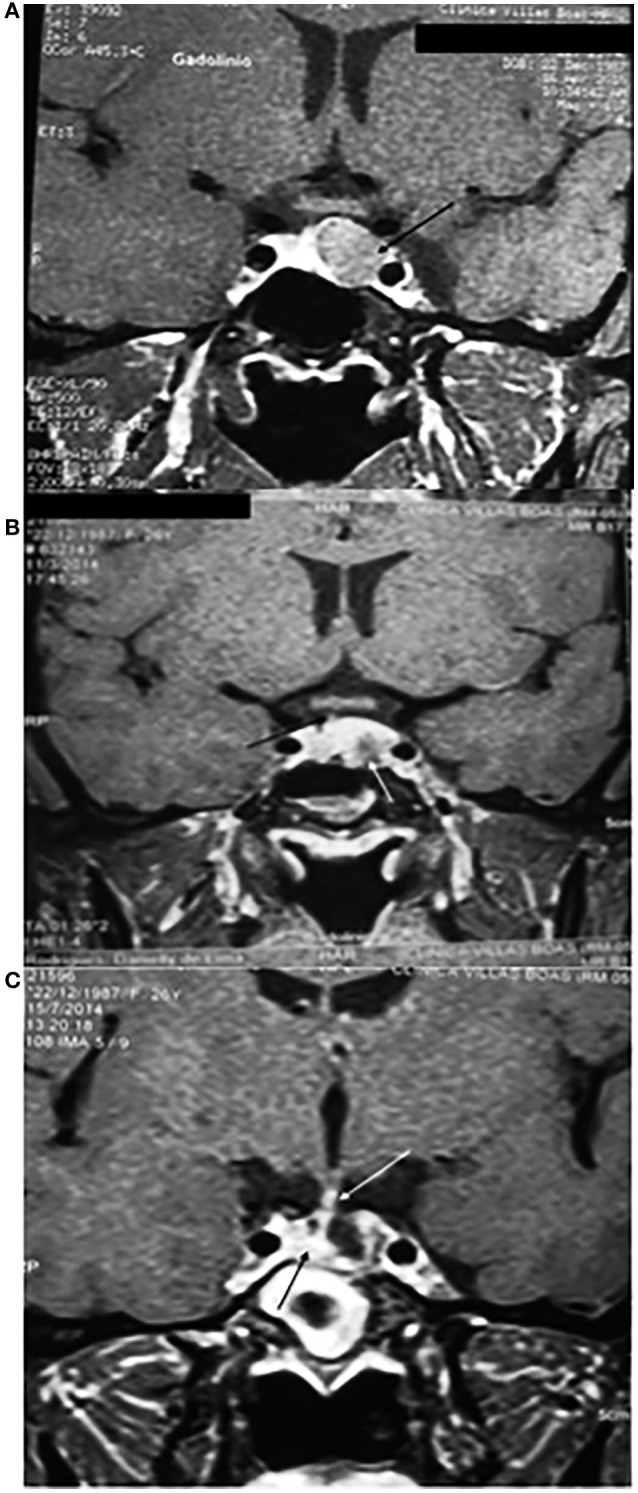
(A) Magnetic resonance imaging of the sella turcica on T1 coronal with contrast before treatment with cabergoline showing a lesion with heterogeneous contrast measuring 1.0 × 1.4 × 1.1 cm, without invasion of the cavernous sinus, but with a close association with the left carotid (black arrow). (B) Imaging performed 47 months after starting the treatment with 2.0 mg per week of cabergoline and 2 months before the transsphenoidal surgery showing the pituitary adenoma with a close association with the left carotid artery (white arrow) and the pituitary stalk diverted to the right (black arrow). (C) Three months after the transsphenoidal surgery, the pituitary adenoma was completely removed with the stalk (white arrow) and the pituitary (black arrow) diverted to the right.
